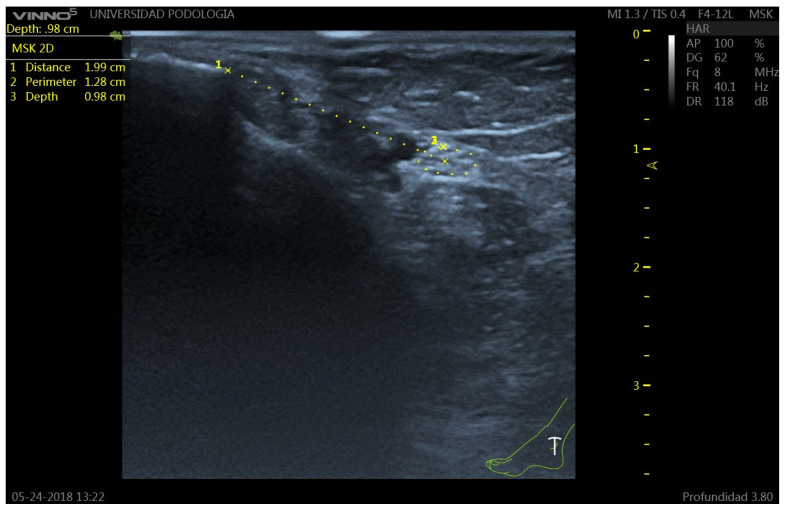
Ultrasound image of the tibial nerve (TN) at the retromalleolar level. The TN appears as a hyperechoic structure with internal hypoechoic fascicles (honeycomb pattern), posterior to the posterior tibial artery (PTA). 1: TN-malleolus distance; 2: TN perimeter; 3: Perpendicular distance from the skin surface to the upper edge of the tibial nerve perimeter.
